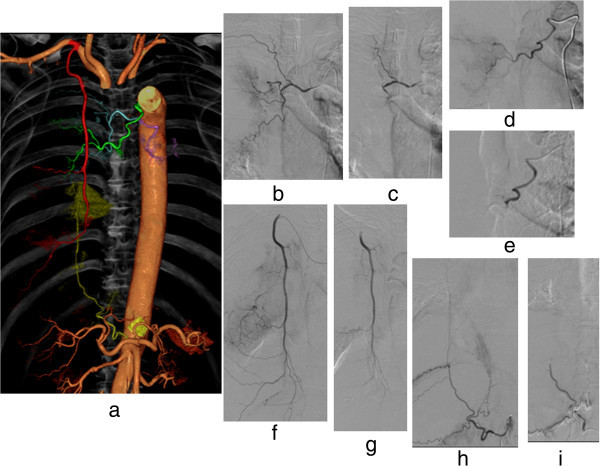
A case of hemoptysis. (a) Hemorrhage-responsible arteriogram created by CT during thoracic aortography depicts the arteries responsible for hemoptysis: the right bronchial artery branching from the thoracic aorta (blue); the right bronchial artery branching from the aortic arch (green); the right inferior phrenic artery branching from the celiac axis (yellow); and the right intrathoracic artery branching from the right subclavian artery (red). Based on this image, embolization with gelatin sponge particles was conducted of each of these arteries: the right bronchial artery branching from the thoracic aorta (b, c); another bronchial artery branching from the aortic arch (d, e); the right intra-thoracic artery branching from the right subclavian artery (f, g); and the right inferior phrenic artery branching from the celiac axis artery (h, i).
